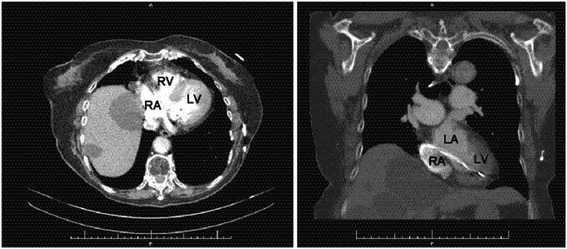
Contrast-enhanced computed tomography showed compression of the right atrium because of a giant liver cyst and the pacemaker lead wire running from the right atrium to the left ventricle. *LA* left atrium, *LV* left ventricle, *RA* right atrium, *RV* right ventricle
